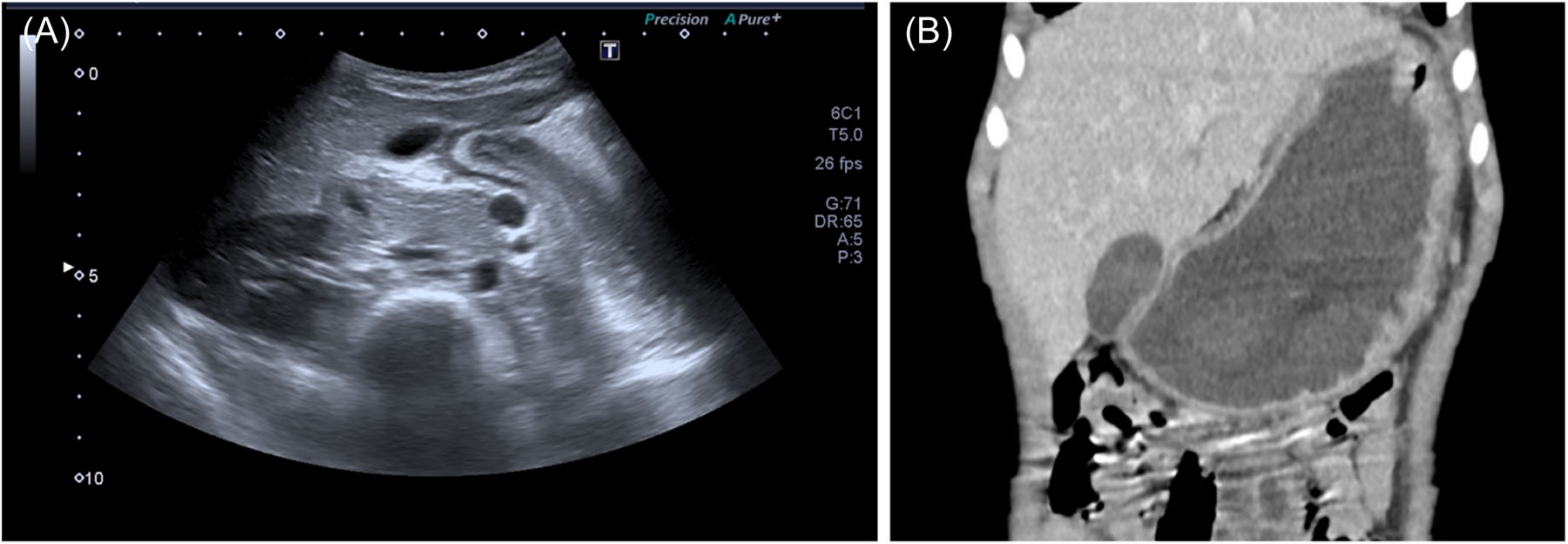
(A) Abdominal ultrasound with pyloric gastric wall thickening at diagnosis. (B) Abdominal CT scan showing gastric dilation, confirming the ultrasound diagnosis.
